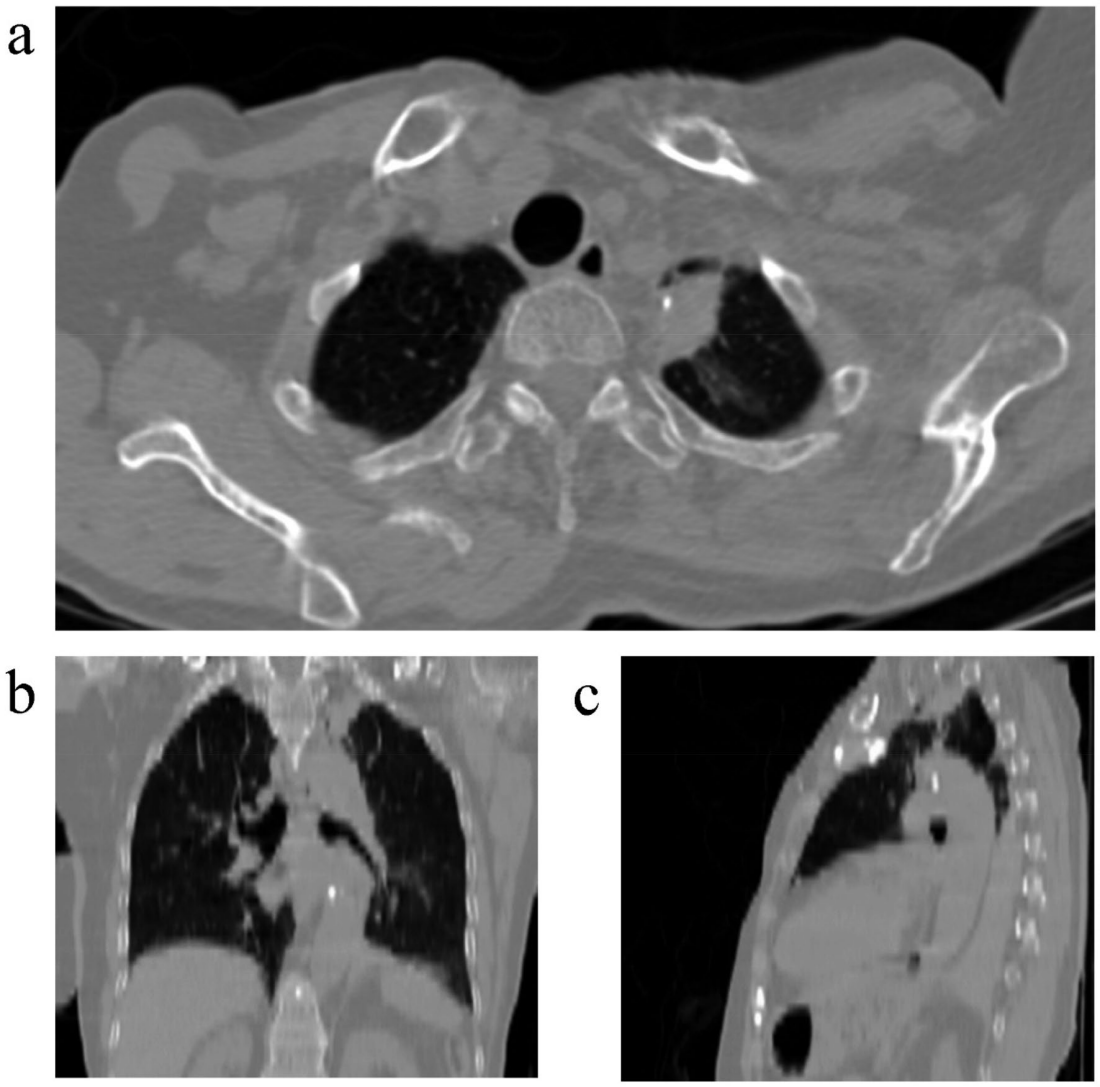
(a) Axial, (b) Coronal, and (c) Sagittal views of CT scan of the pneumoconiosis lesion in the superior left lung lobe, showing a mildly spiculated border with a small area of peripheral calcification.
